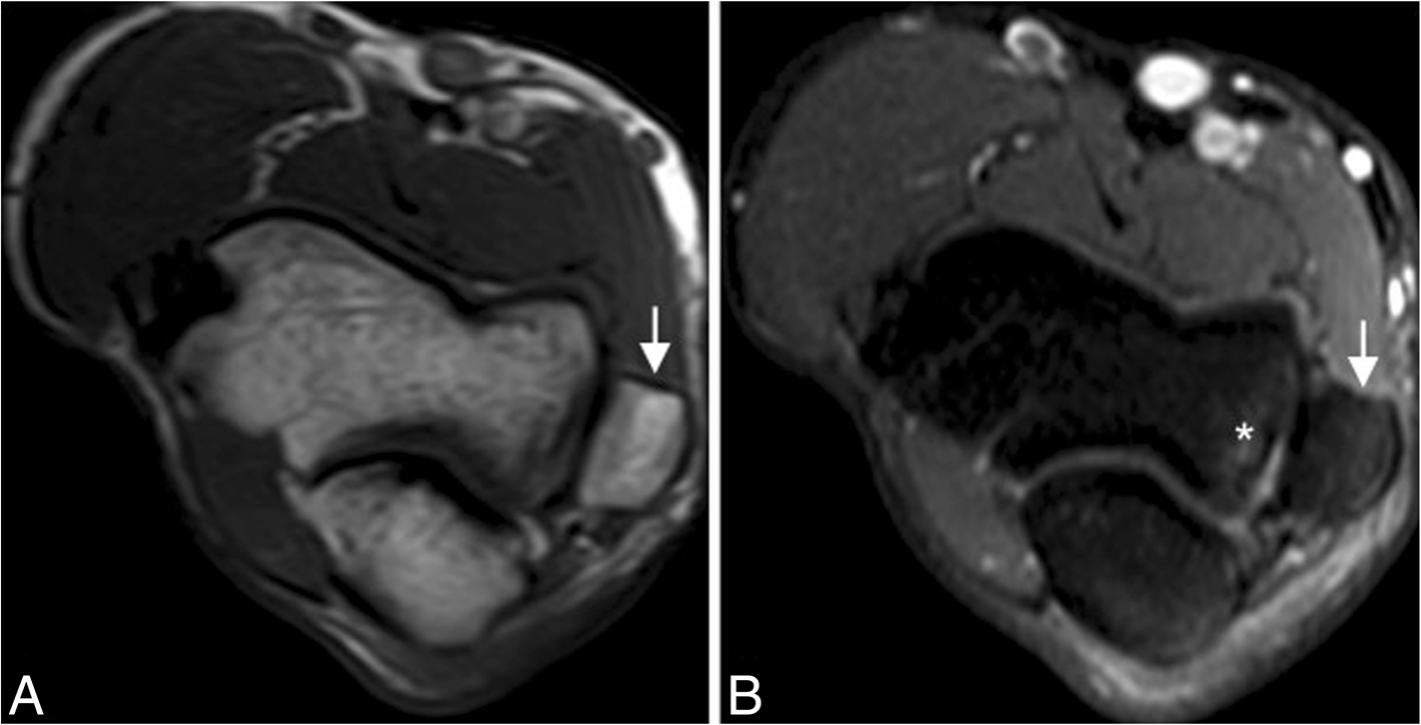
A 50-year-old man with elbow pain and decreased range of motion due to an apophyseal injury sustained before physeal closure. Axial T1-weighted MRI (a) and axial FS PD-weighted MRI (b) showing chronic non-union fracture of the medial epicondyle and heterotopic ossification along the anterior band of the medial collateral ligament (white arrows). Note stress reaction, manifest as bone marrow edema (white asterisk)
